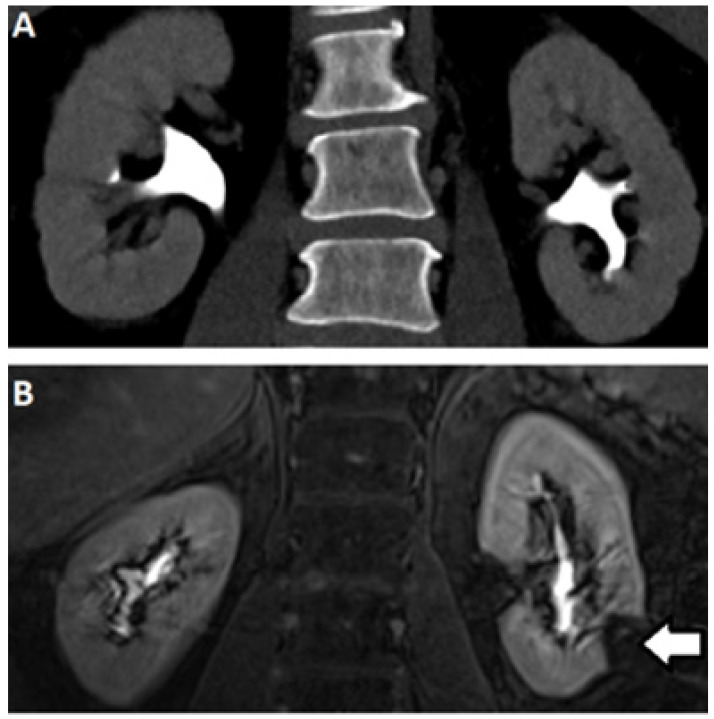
Excretory phase CT (A) and MRI (B) demonstrating normal caliber renal collecting systems without filling defect. Note the absence of signal in the left lower pole (arrow) corresponding to a renal cyst.
